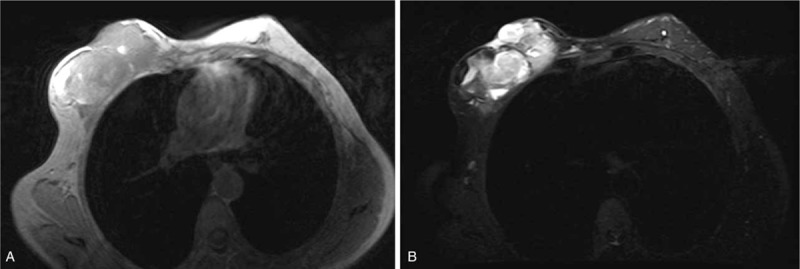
Magnetic resonance imaging findings. A,B Magnetic resonance images showing a large tumor in the right breast.
